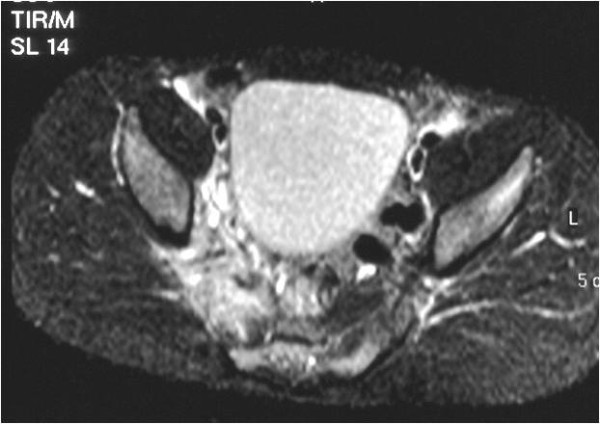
Axial short tau inversion recovery (STIR) image; MRI scan taken at the time of antibiotic treatment initiation. The right piriformis muscle is enlarged, bulging anteriorly, with high signal intensity within and loss of definition of its muscle striations, consistent with edema. Compare to the normal piriformis muscle on the left.
